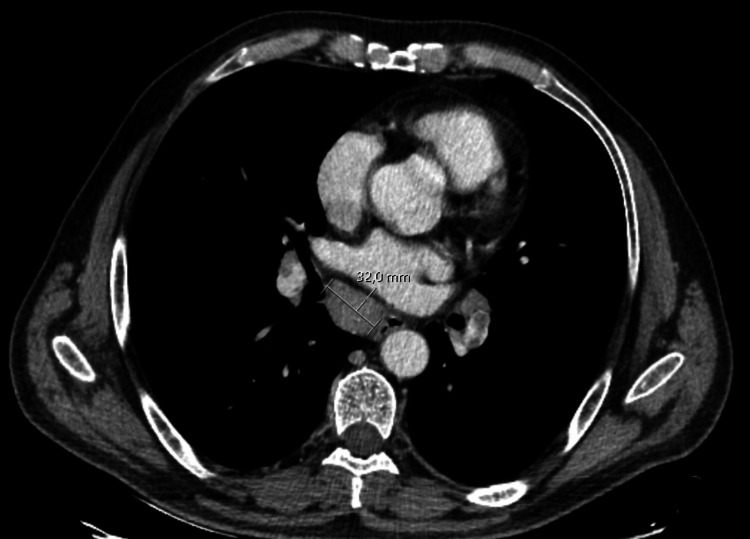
Thoracic CT imaging Computer tomography imaging of the thorax, showing lymphadenopathies at the base of the trachea measuring 32 mm × 23 mm
